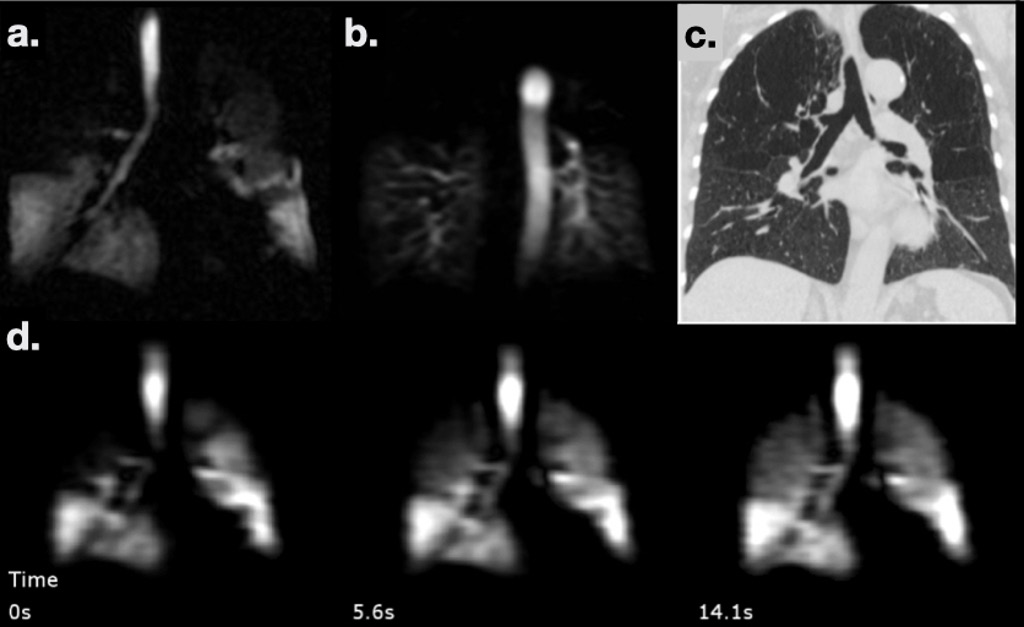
Patient F: LVRS case study. 59-year-old ex-smoker with GOLD Stage 3 COPD, with significant limitation to daily life and severe hyperinflation. The patient had completed pulmonary rehabilitation and was under consideration for lung volume reduction with endobronchial valves and a complementary HP gas MRI and CT clinical work-up was requested. a. HP ^3^He ventilation MRI, showing no ventilation in the right upper lobe and reduced ventilation in the left upper lobe. b. Dynamic contrast enhanced perfusion MRI, showing no perfusion in the upper lobes. C. Unenhanced CT image, indicating upper lobe-predominant bullous emphysema. D. ^3^He delayed ventilation images (three time-points during the same breath-hold), showing wash-in of MRI signal in the right upper lobe over time, consistent with collateral ventilation, which was confirmed by Chartis (gas catheter bronchoscopy). Based on these images, a decision was made to insert endobronchial valves into the left upper lobe and successful lobar collapse was seen post-procedure. After the procedure, the patient reported a significant improvement in symptoms and was able to perform the usual activities of daily living. The complementary use of multimodality imaging in the work-up for this LVRS candidate helped to prevent a likely unsuccessful right-sided procedure and provided reassurance that the left upper lobe was a viable target. COPD, chronic obstructive pulmonary disease; LVRS, lung volume reduction surgery.
